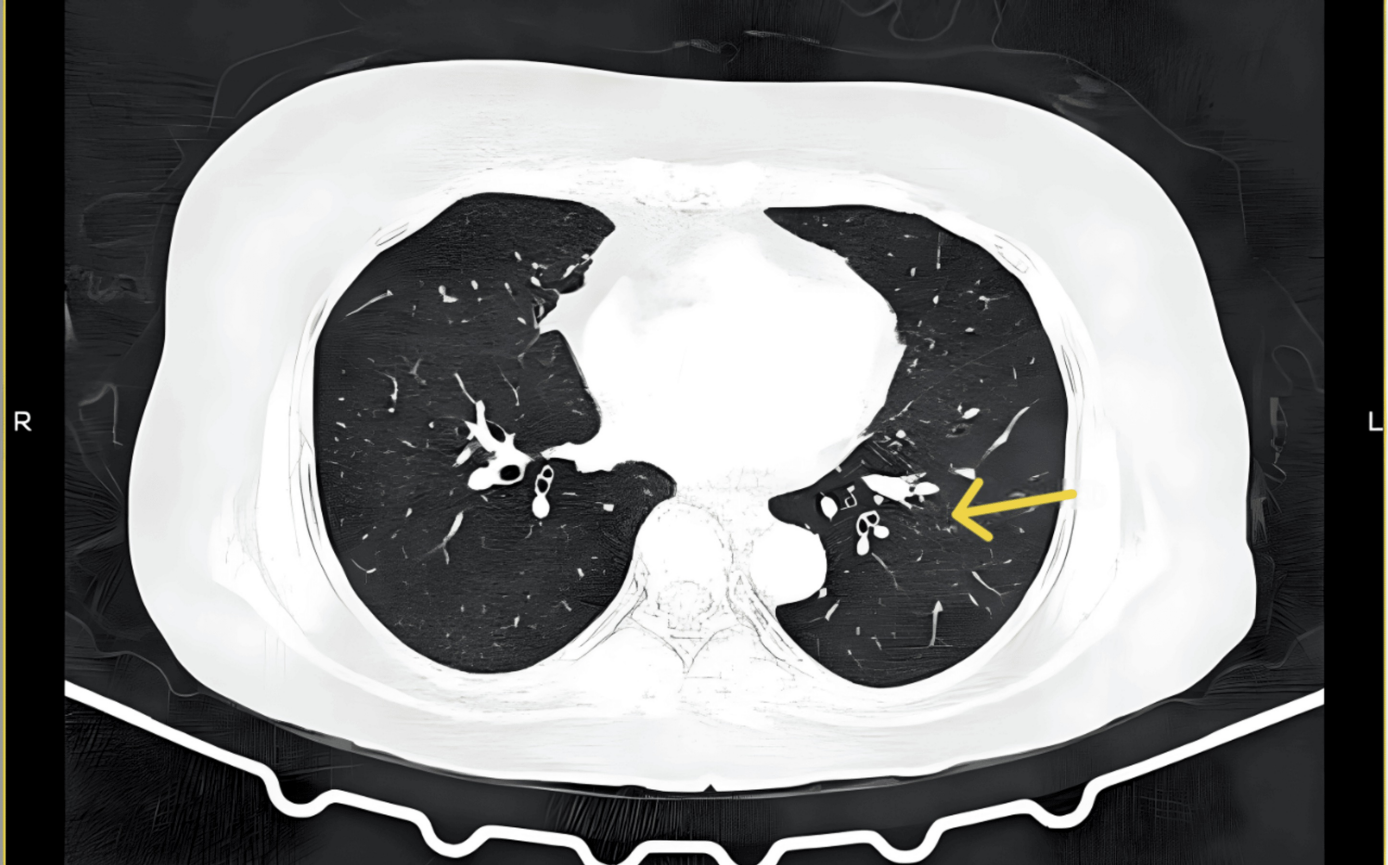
CT of the thorax. CT of the thorax shows few fibrotic strands with subtle ground-glass opacity in the left lower lobe, with normal trachea, bronchi, heart, and mediastinal vessels.
